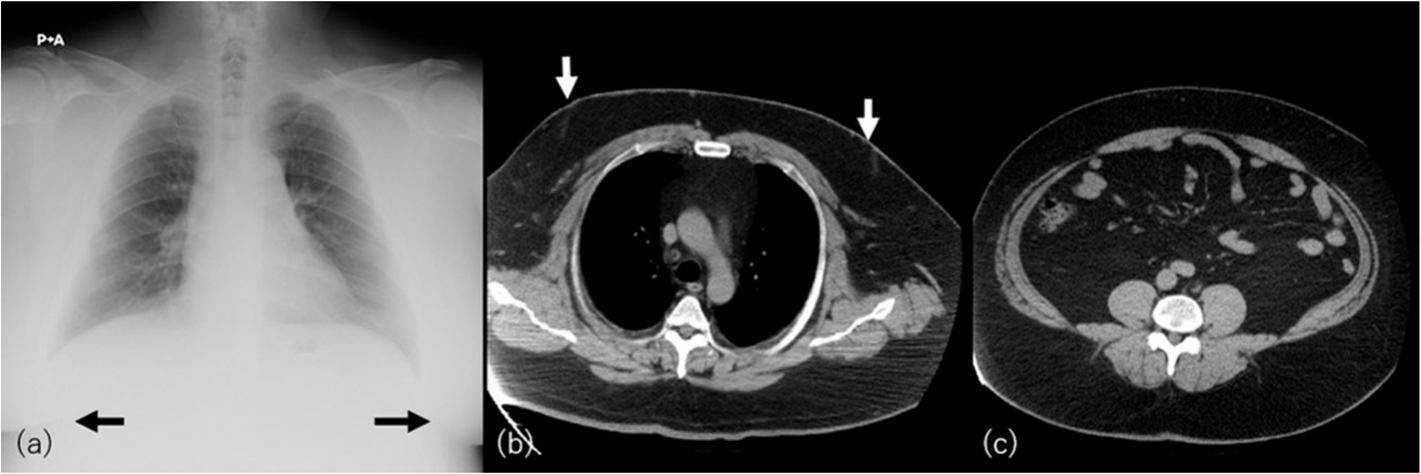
A 37-year-old man with binge eating disorder and overeating habitus (BMI = 53.2). a Chest radiograph shows bilateral pseudogynecomastia (black arrows). b CT of the chest and (c) abdomen shows increase of subcutaneous and visceral adipose tissue. Note the absence of extensive growth of mammary gland but pure fat deposition on the breast (white arrows)
